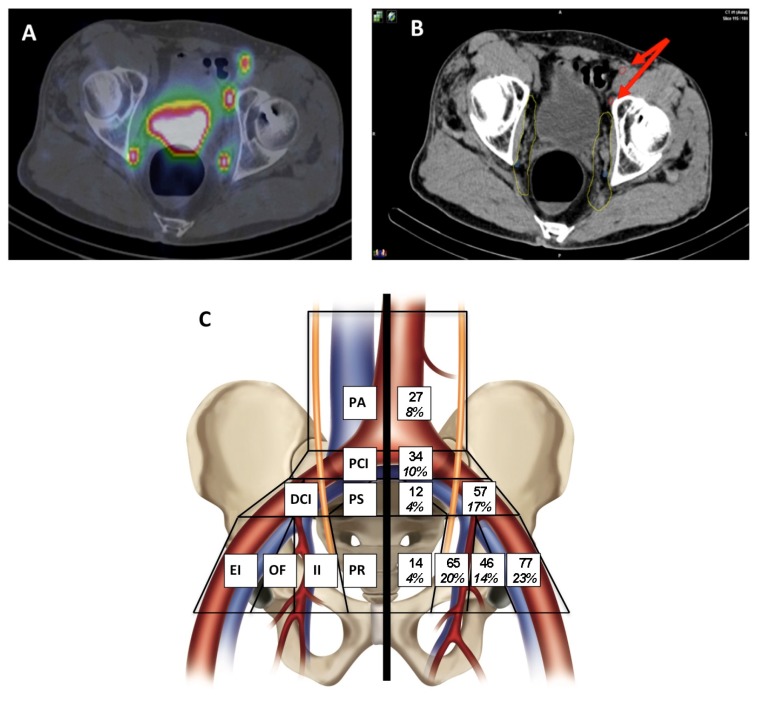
Anatomical distribution of sentinel lymph nodes (SLN) by lymphoscintigraphy (SPECT/CT) in different abdomino-pelvic regions. PA: para-aortic; PCI: proximal common iliac (between aortic bifurcation and L5-S1 interspace); DCI: distal common iliac (between L5-S1 interspace and iliac bifurcation); PS: pre-sacral; EI: external iliac; OF: obturator fossa; II: internal iliac; PR: peri-rectal. (A) Representative image of an axial lymphoscintigraphy (SPECT/CT); (B) two left- and right-sided II SLN are located within the RTOG clinical target volume (CTV) (yellow) and two left-sided EI SLNs are located outside the RTOGCTV N (red circles and red arrows); (C) distribution of sentinel lymph nodes.
